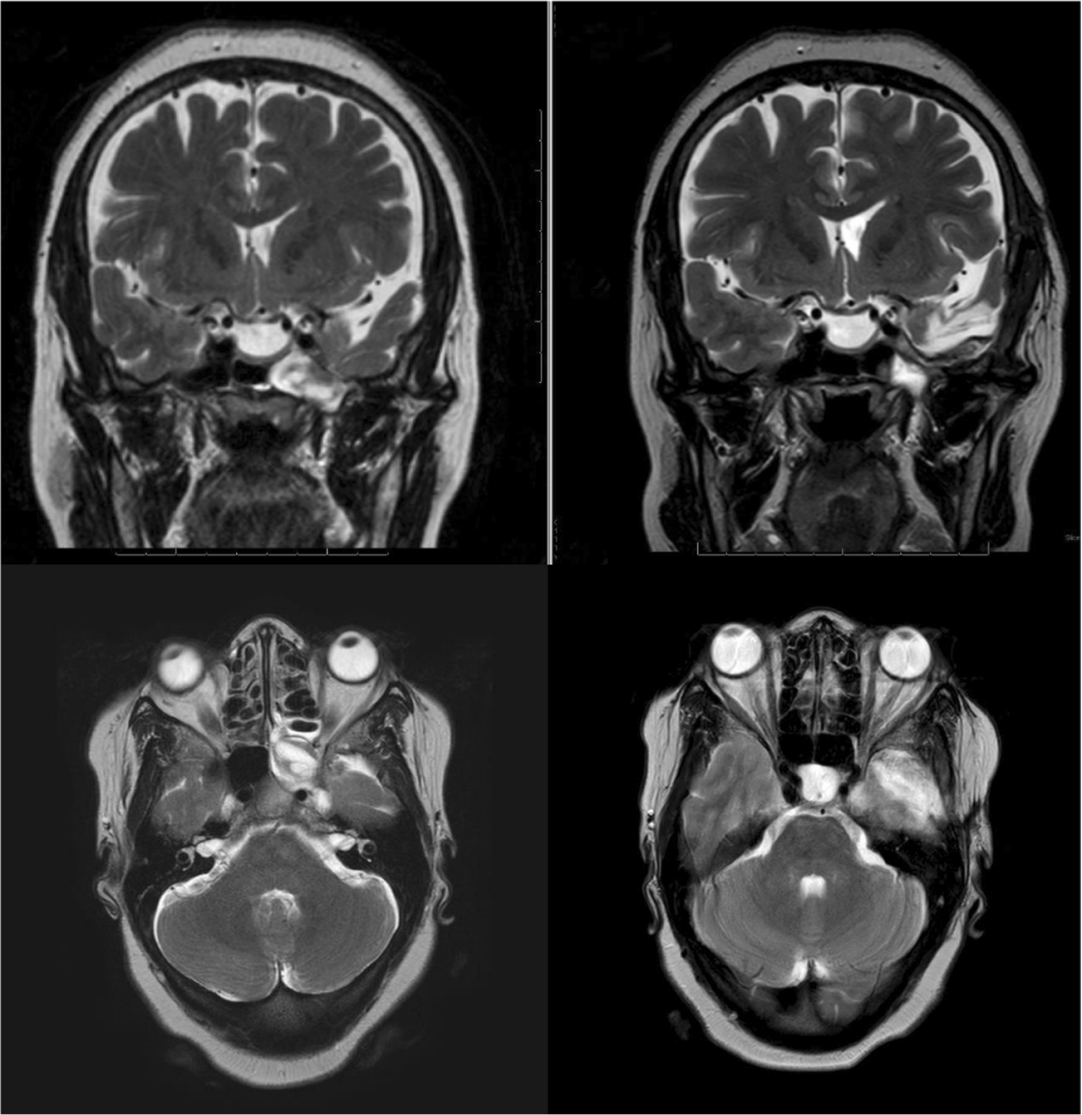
T2-weighted MRI of the brain. Her pre-operative imaging is on the* left*. On the* right*, her post-operative imaging is shown. The dysplastic temporal pole has been resected and a plaque of fatty material is visible within the encephalocele's former cavity
